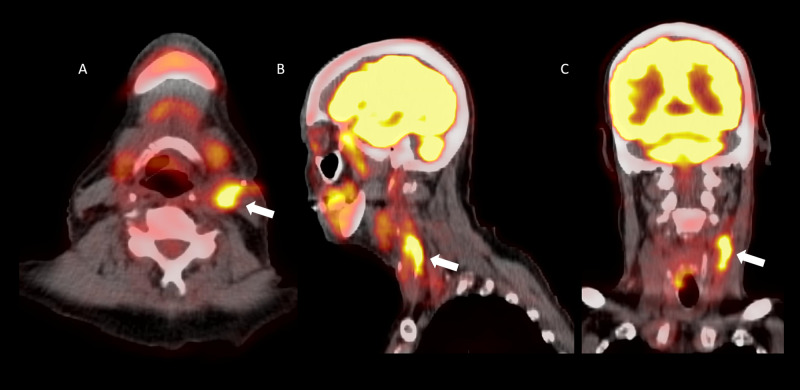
Axial (A), sagittal (B), and coronal (C) positron emission tomography/computed tomography fusion images demonstrating fluorodeoxyglucose uptake in the region of the operative bed (white arrows).
